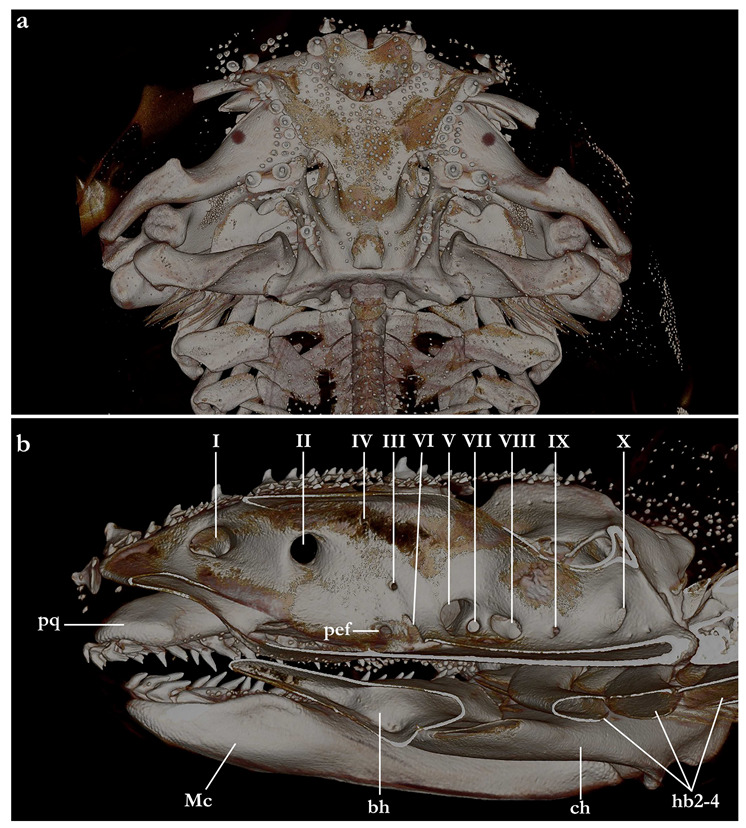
Squatina africana*, ZMH 123064, juvenile female, 309 mm TL; micro-computed tomography scans of the skull: (a) dorsal view, (b) sagittal cross-section. Abbreviations: bh, basihyal; ch, ceratohyal; hb2-4, hypobranchials 2–4; Mc, Meckel’s cartilage; pef, pseudobranchial artery foramen; pq, palatoquadrate; I, olfactory bulb foramen; II, optic nerve foramen; III, oculomotor nerve foramen; IV, trochlear nerve foramen; V, trigeminal nerve foramen; VI, abducens nerve foramen; VII, facialis nerve foramen; VIII, auditory nerve foramen; IX, glossopharyngeal nerve foramen; X, vagus nerve foramen. Anterior to top in (a) and left in (b).
